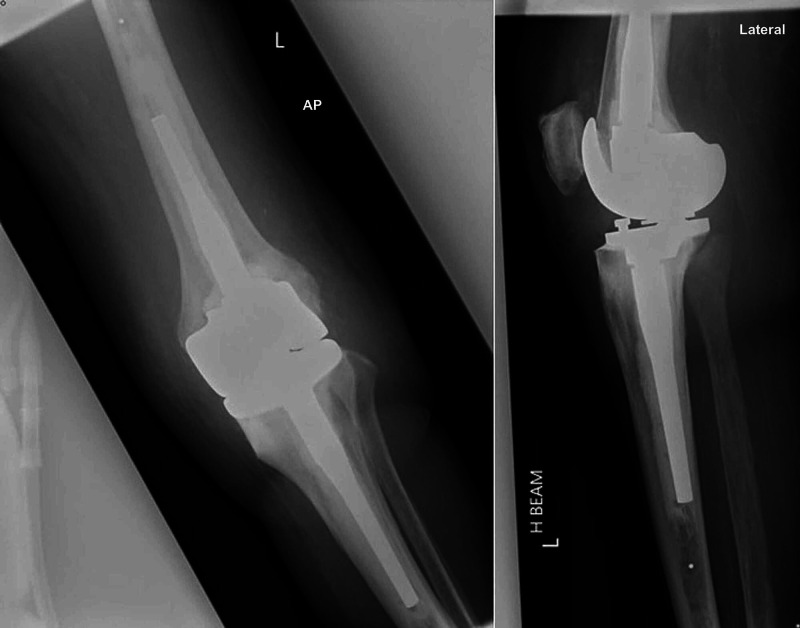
Anterior-posterior and lateral radiographs of the left knee post-TKA using a rotating hinge endolink. TKA, total knee arthroplasty
